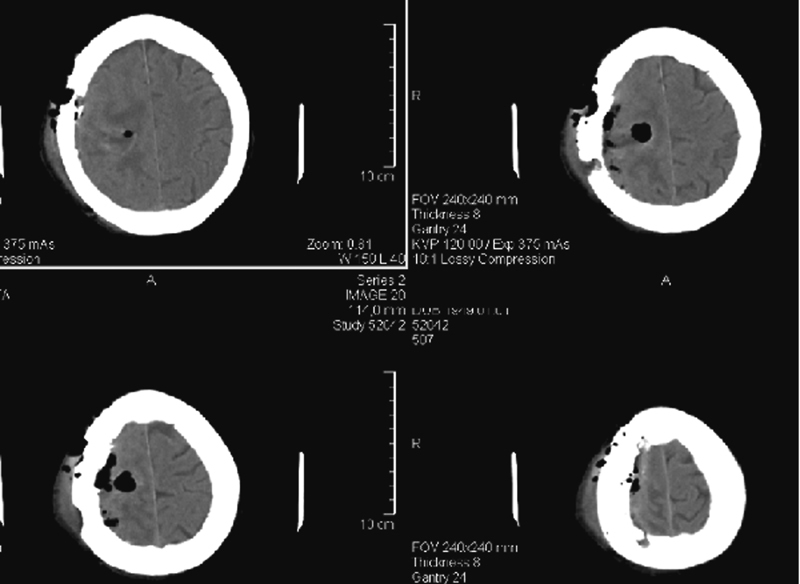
Postoperative computed tomography. This image shows the radical removal of the lesion.
